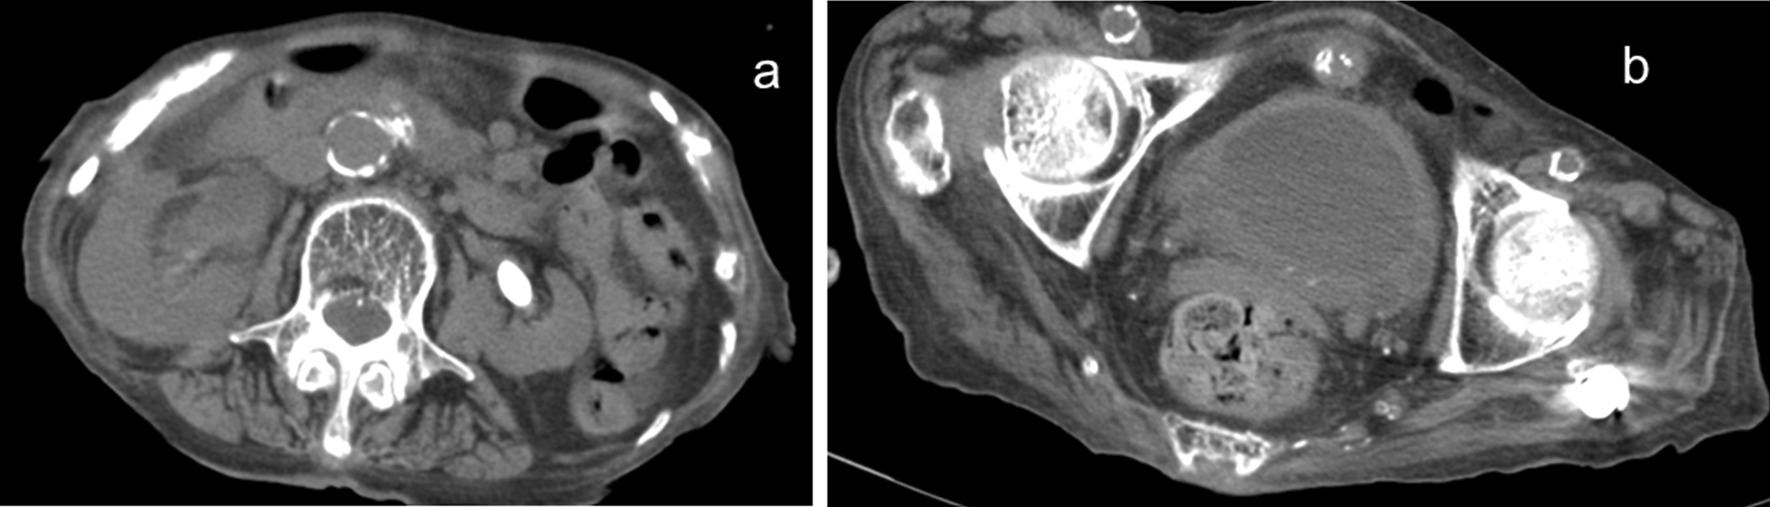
Abdominal computed tomography at admission. There are stones in both kidneys, right ureteral dilation (a), and a thickened bladder wall (b)
